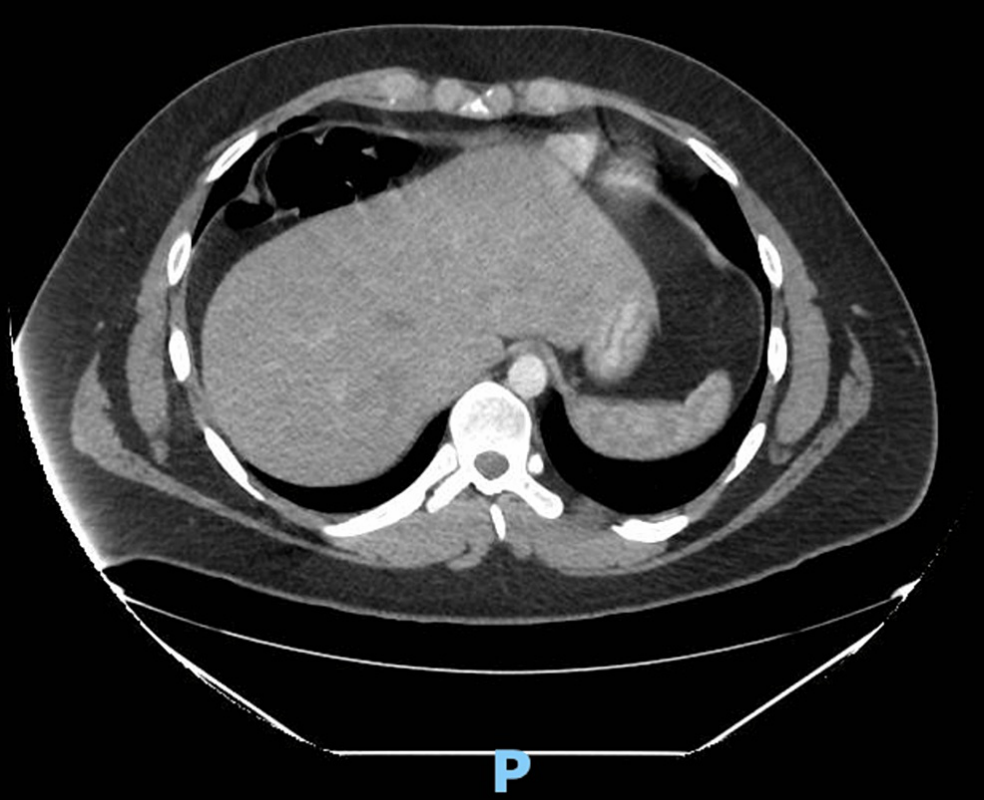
CT of Right Colonic Interposition
